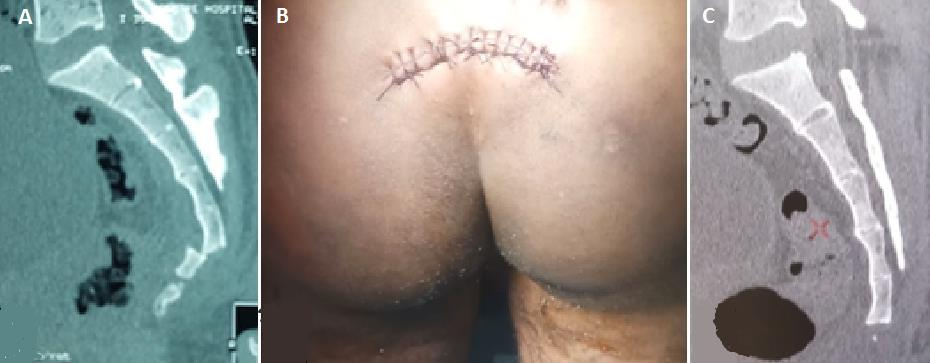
(A) sacro-coccygeal CT scan showing in sagittal section, a dislocation of the entire coccyx compared to the 5^th^ sacred piece; (B) surgical approach; (C) an excision of the coccyx
